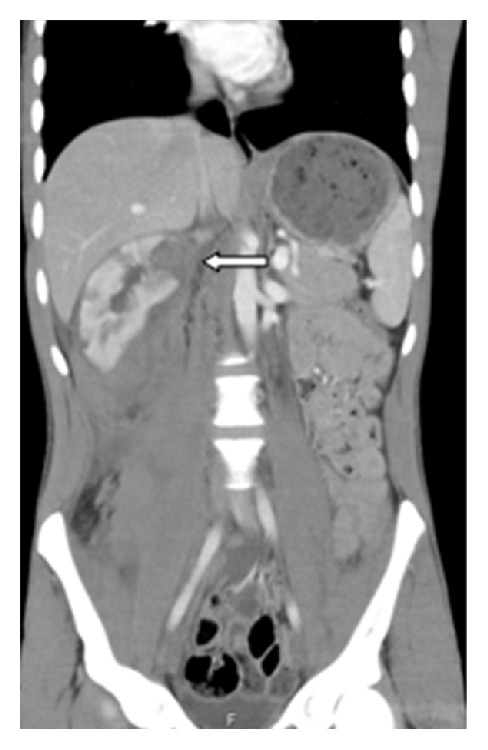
Contrast enhanced computed tomography of the patient where multiple infarcts can be seen in right kidney (arrows).
